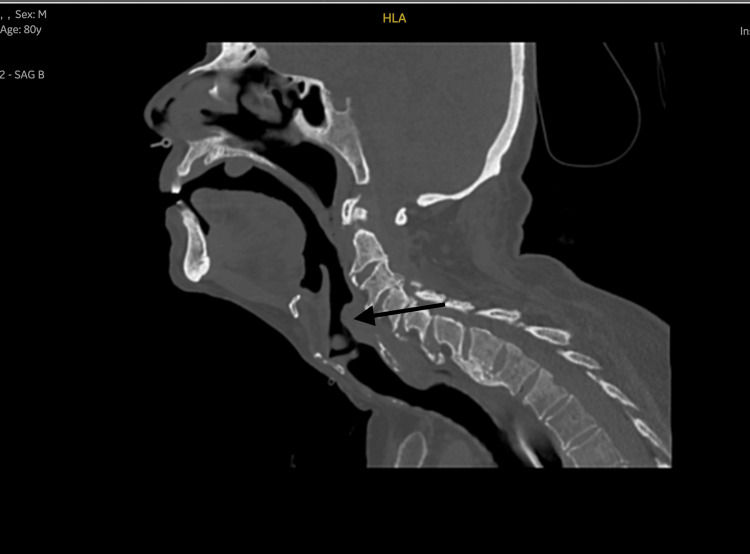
Post-rupture CT scan of the neck, sagittal view, showing resolution of the hypopharyngeal cyst (black arrow) and re-expansion of the airway
